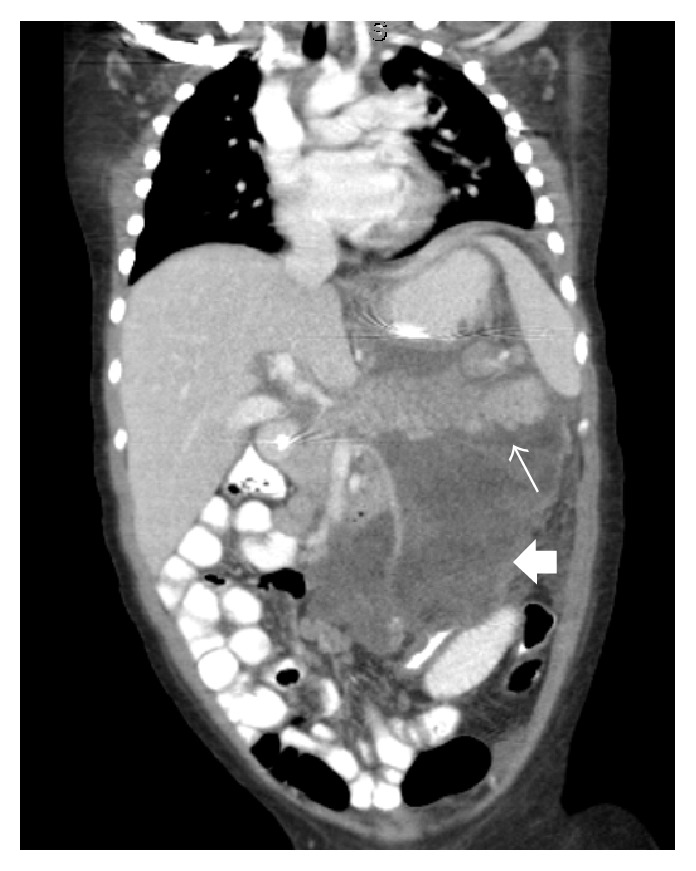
Abdominal CT (coronal view): 10 cm × 8.4 cm left-sided peritoneal mass (broad arrow) with significant inflammation of the pancreas (thin arrow).
